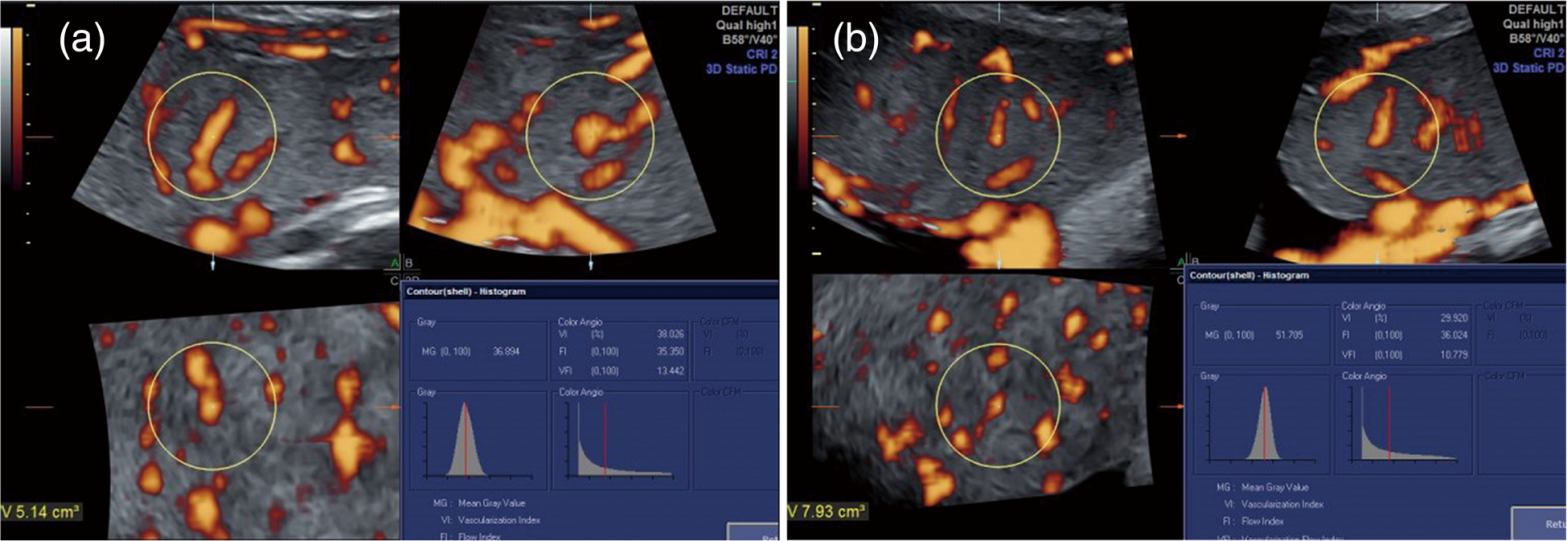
Acquisition of placental volumes with three-dimensional power Doppler in twin pregnancy. The volume data was acquired close to the umbilical cord implantation site of a normal monochorionic diamniotic twin pregnancy (fetus a and fetus b) at gestational week 32^+ 6^. The sphere volume (yellow sphere frame) varied according to placental thickness. Placental indices of fetus a: volume 5.14 cm^3^; VI 38.026; FI 35.35; VFI 13.442. Placental indices of fetus b: volume 7.93 cm^3^; VI 29.92; FI 36.024; VFI 10.779. FI, Flow index; VFI, Vascularizationflow index; VI, Vascularization index
